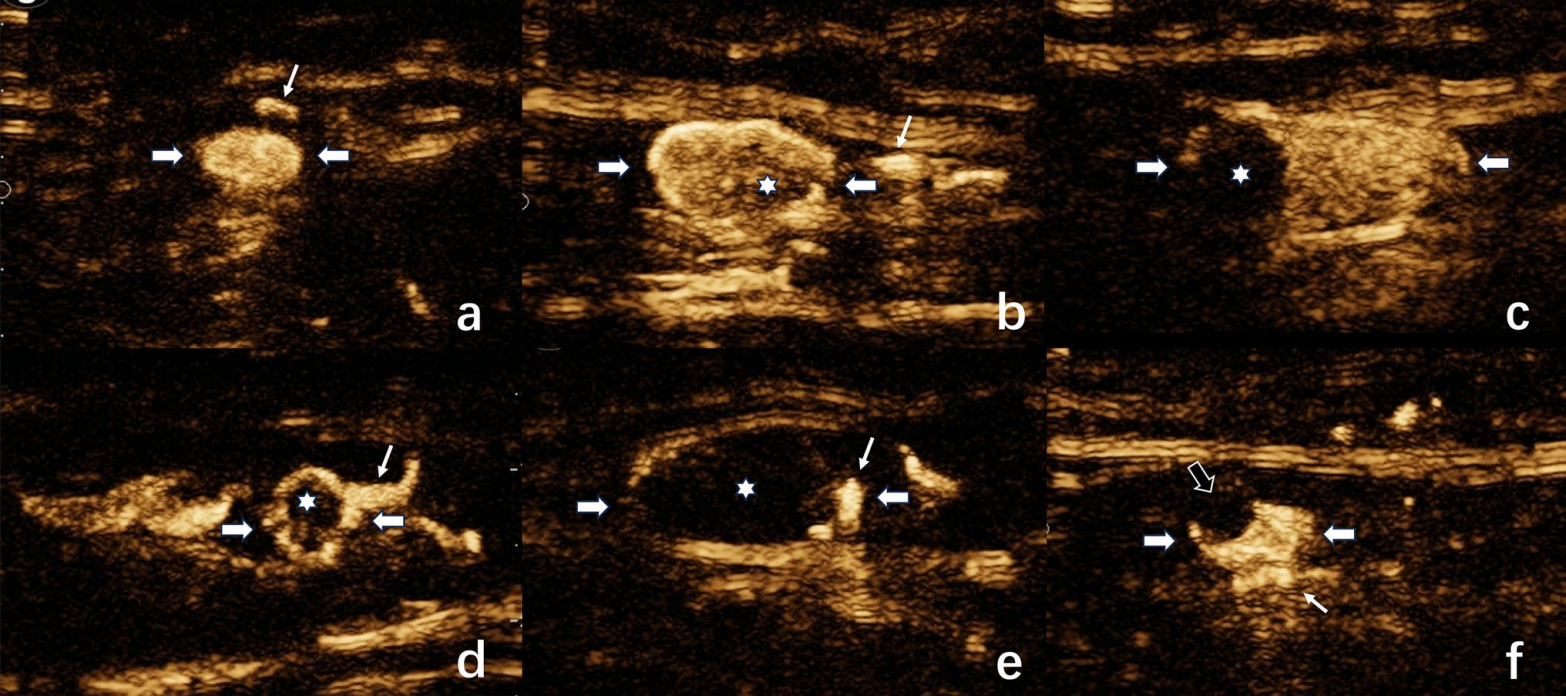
Enhancement characteristics of different types of SLNs on L-CEUS. a Type I: overall bright homogeneous enhancement throughout the SLNs (white thick arrow). b Type II: homogeneous enhancement of the cortex and uniform distribution of contrast agent (white asterisk) in the cortical area of lymph nodes. c Type III: uneven distribution of contrast agent in the cortical area of lymph nodes or the appearance of nonenhanced areas (white asterisk). d Type IV: peripheral complete or incomplete annular enhancement. e Type V: no contrast agent (white asterisk) enters the lymph node through the end of the enhanced afferent lymphatic vessels (white thin arrow). f The presence of filling defects (black arrow) in SLNs. Sentinel lymph nodes (white thick arrow), uneven enhancement or no enhancement (white asterisk), lymphatic vessels (white thin arrow), filling defects (black arrow). *SLNs* sentinel lymph nodes; *L-CEUS* lymphatic contrast-enhanced ultrasound
